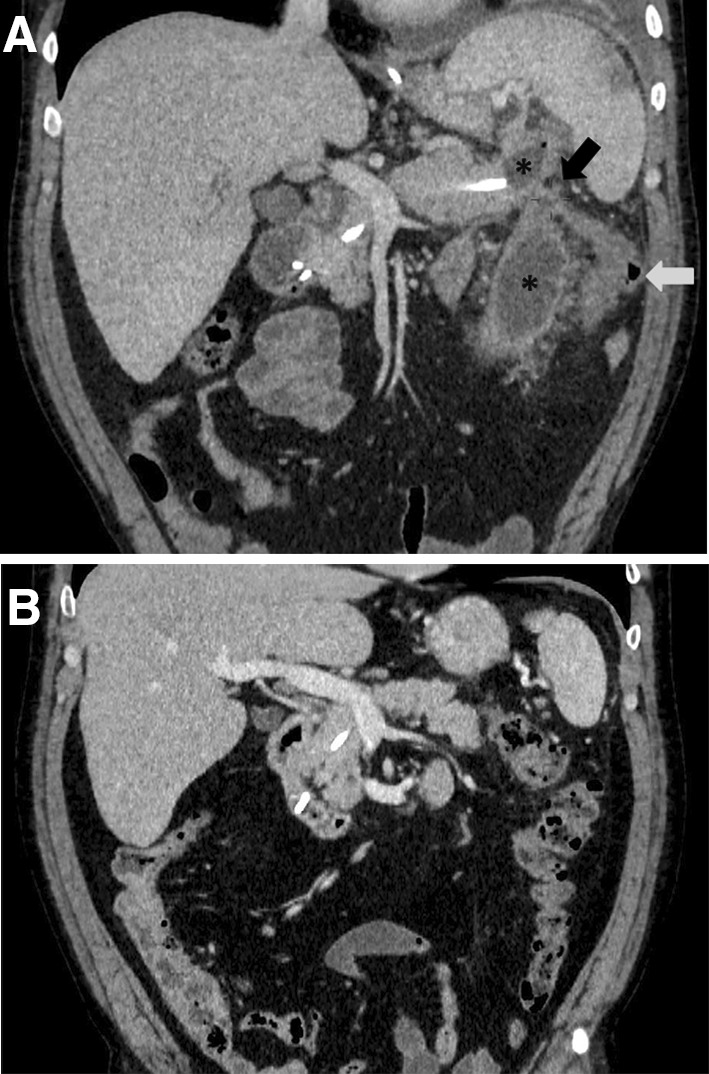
A Abdominal contrast-enhanced computed tomography (CECT) done during the endoscopic treatment (video 1) showed a pancreaticocolonic fistula (red arrow) between the walled-off pancreatic necrosis cavity (blue stars) and the colon lumen (green arrow) in the area of splenic flexure. Nasal drain 7 French along with pancreatic endoprosthesis 7 French was inserted to the main pancreatic duct through the major duodenal papilla (active transpapillary drainage). (Color figure online)
